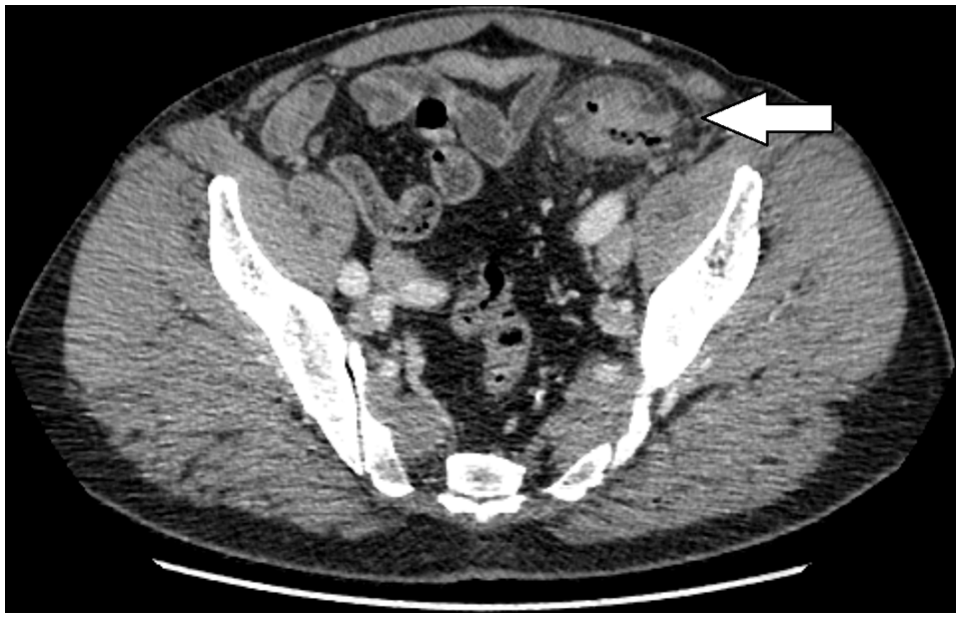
A patient with lower left abdominal pain. This computed tomography scan of the lower abdomen shows colonic wall thickening in the proximal sigmoid colon, diverticula (arrow), and pericolic fat stranding. A diagnosis of uncomplicated diverticulitis was made.
